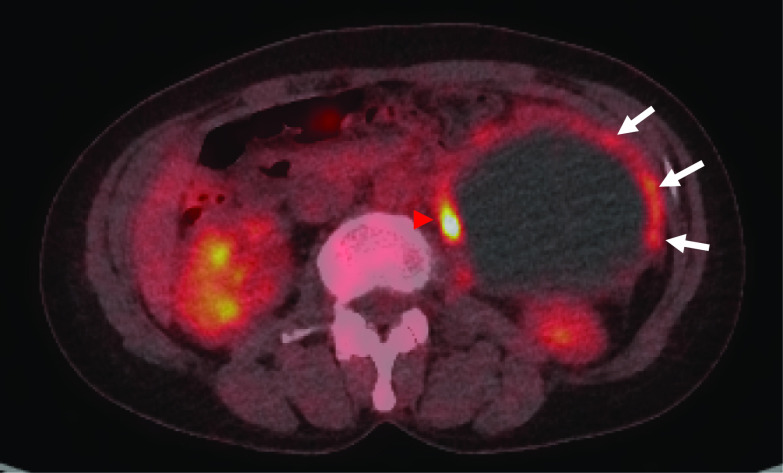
Preoperative 18 F-fluorodeoxyglucose (FDG)-positron emission tomography/computed tomography (PET/CT) findings. FDG-PET/CT revealed mottled FDG uptake in the whole cyst wall (white arrows) and intense FDG uptake of several mural nodules (red arrow’s head)
